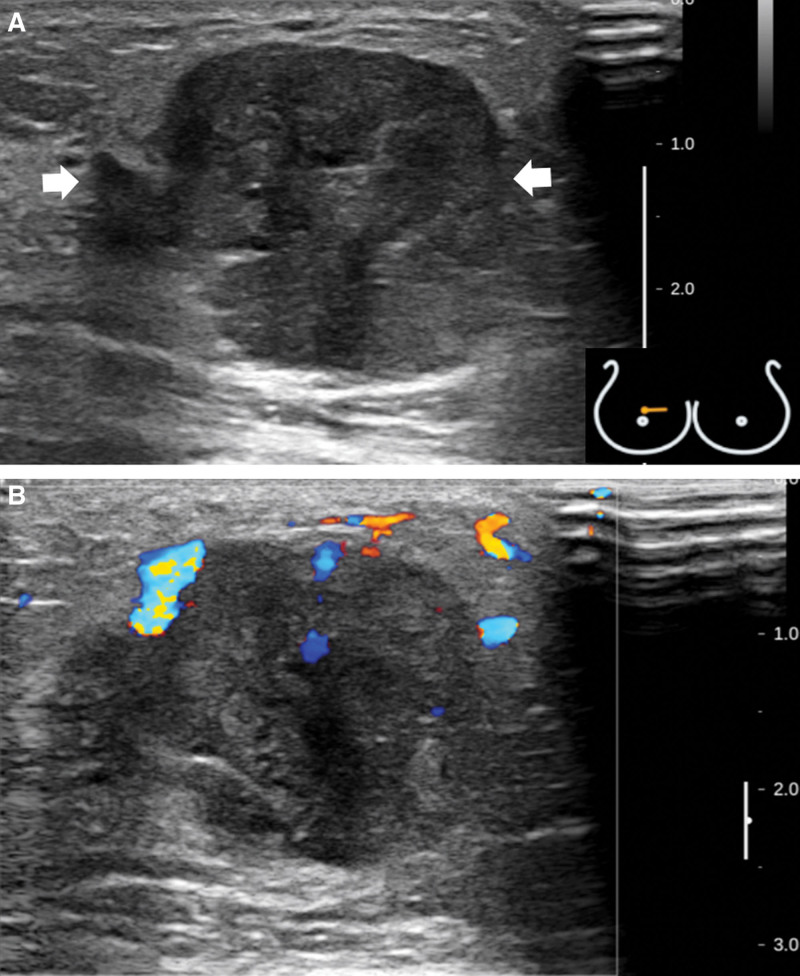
(A) A 3.4 × 2.3 cm irregularand heterogeneous echoic mass (arrows) with posterior acoustic enhancement at 2 o’clock of the right breast is revealed via ultrasonography. (B) Internal vascularity identified in the mass on color Doppler imaging. The lesion is categorized as Breast Imaging Reporting and Data System category 4c.
